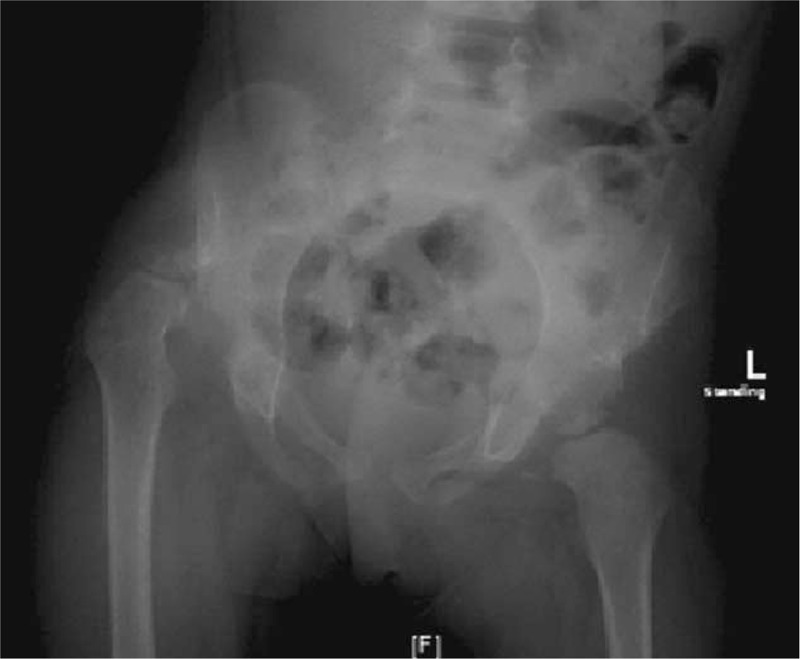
Twenty-month-old girl with bilateral developmental dysplasia of the hips: Anteroposterior radiograph of the pelvis showing complete dislocation of the right hip.
